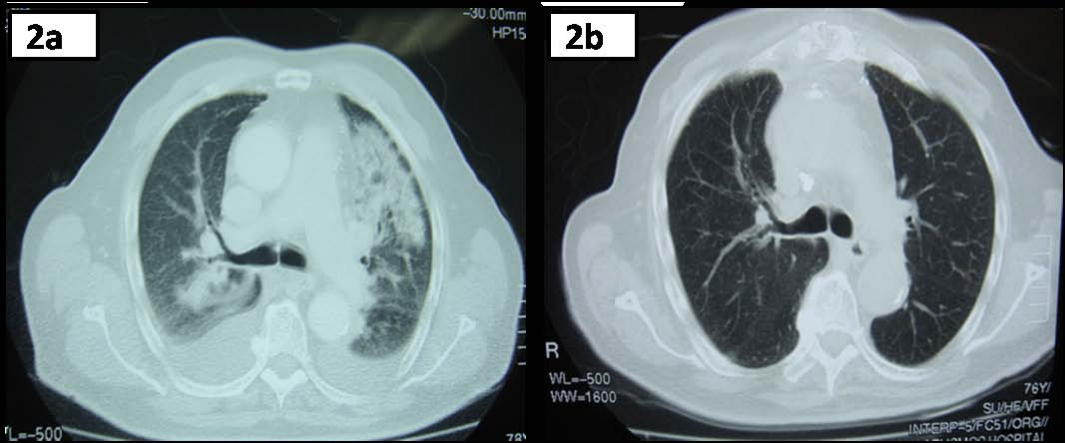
Chest HRCT-scans. a. Chest HRCT-scan demonstrating bilateral irregularly shaped nodular consolidations with air bronchograms and bilateral pleural effusions. b. Chest HRCT-scan, one month after daptomycin discontinuation, demonstrating complete resolve of nodular consolidations and bilateral effusions.
